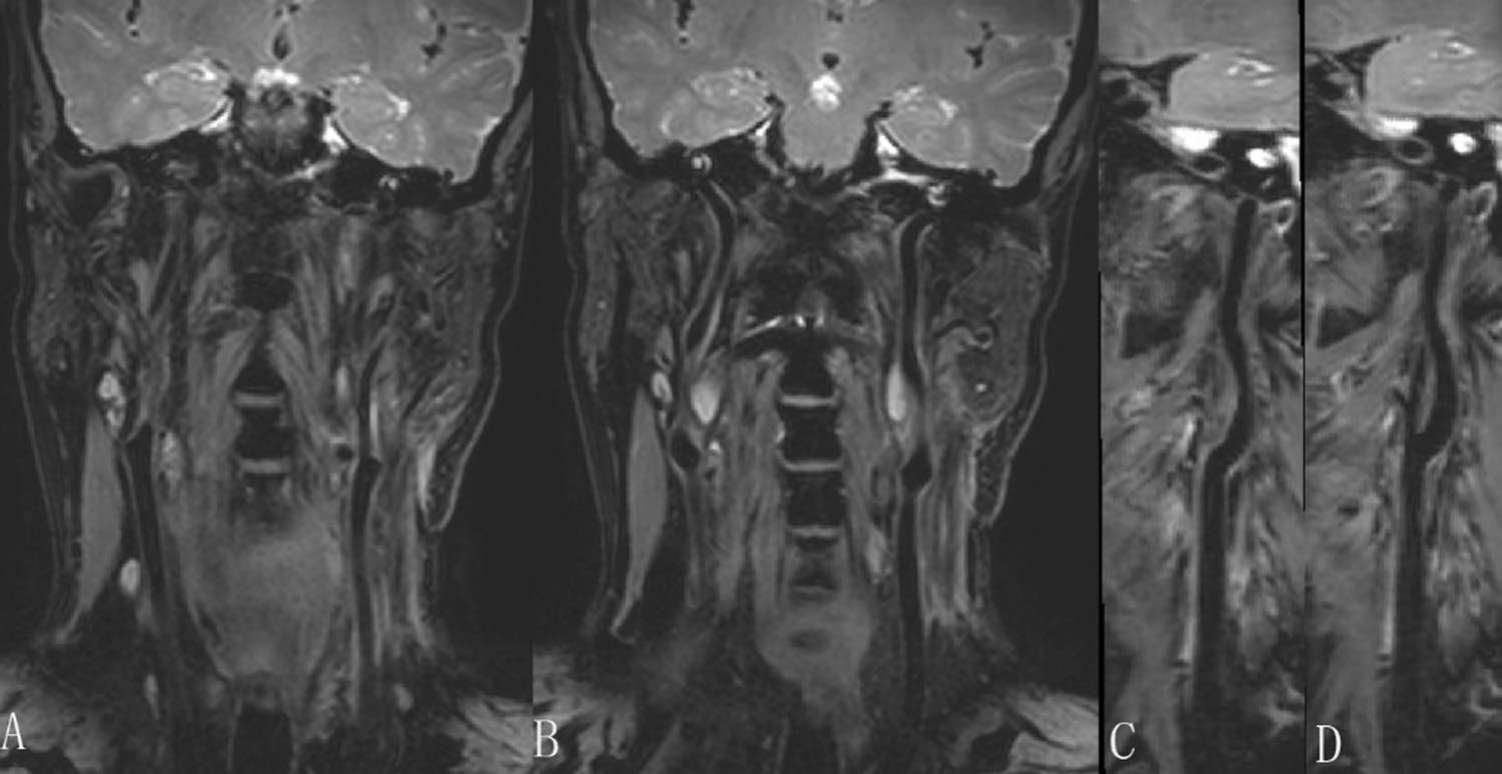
The carotid artery wall in the neck area were well displayed on 3D T2-weighted fast field echo. (A,B) coronal view, and (C,D) sagittal view.
